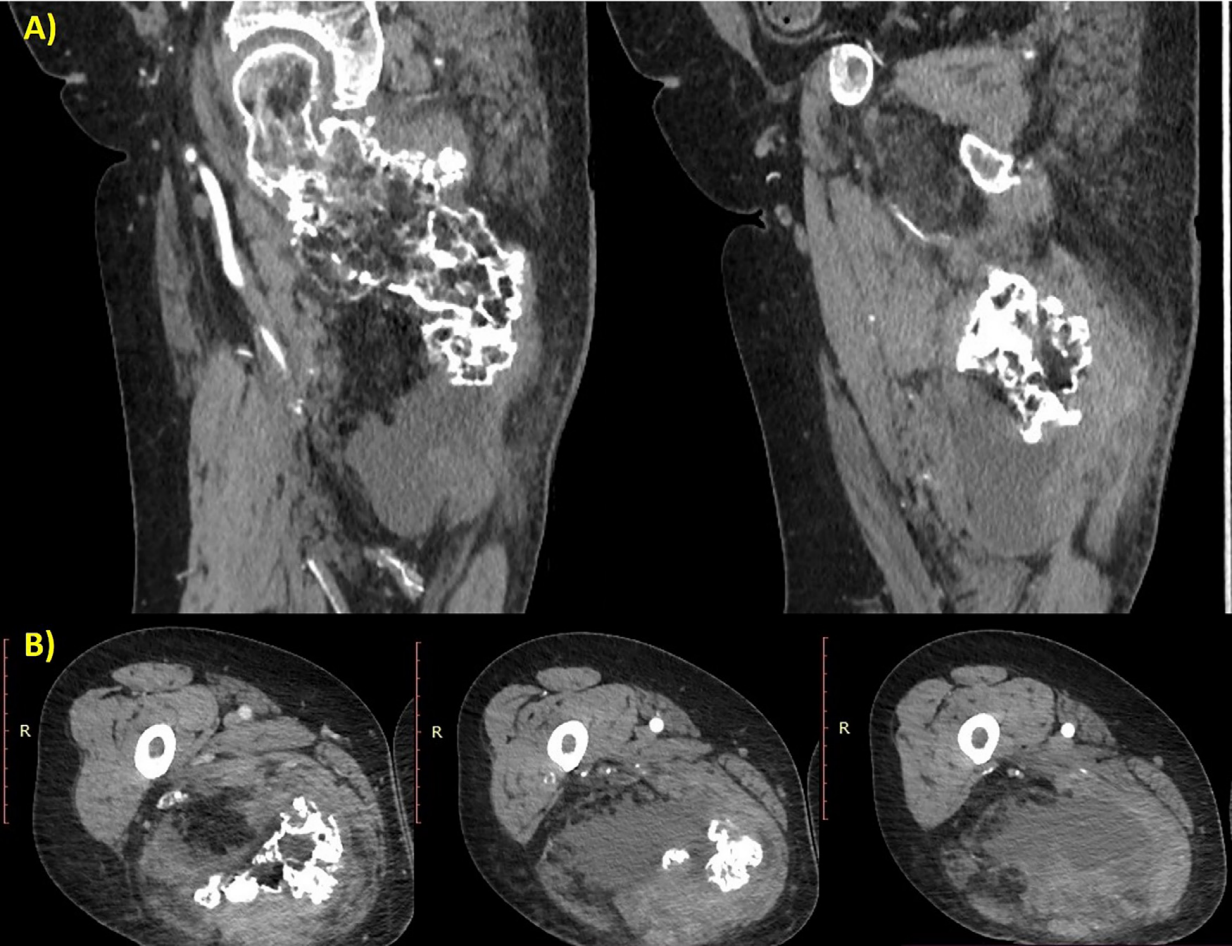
(A, B) Sequential CT scan performed after contrast agent administration in sagittal (A) and axial plane (B). In the lower part, the soft tissues were involved and showed heterogeneous density with fluid components and slight contrast enhancement. There was marked thickening of the muscle bundles of the quadratus and biceps femoris.
